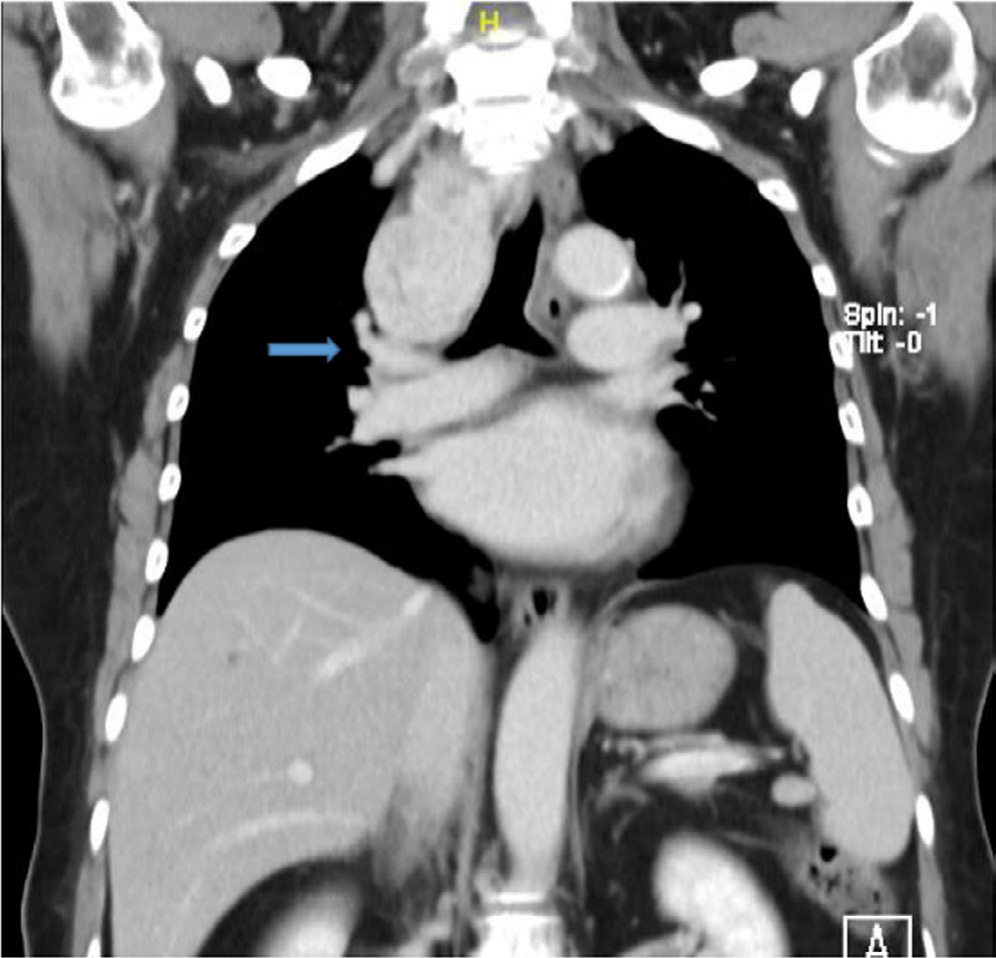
Coronal section of the CT scan
